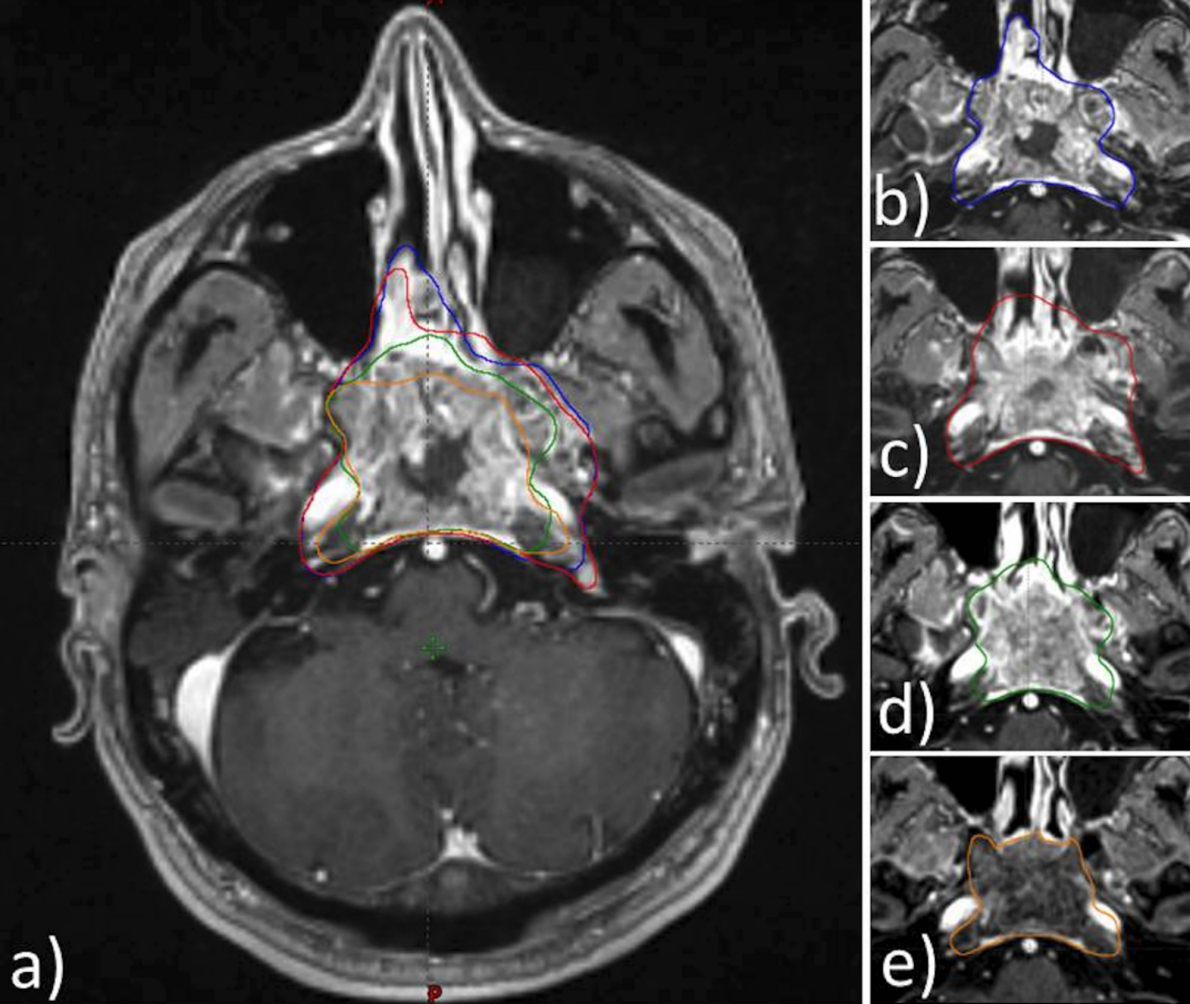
A representative set of axial MRIs acquired for Patient 2 that clearly demonstrates GTV reduction at each time point. a) All time points; b) GTVp_0_; c) GTVp_1_; d) GTVp_2_; e) GTVp_3_ MRI: magnetic resonance imaging; GTV: gross tumor volume; GTVp: primary gross tumor volume
